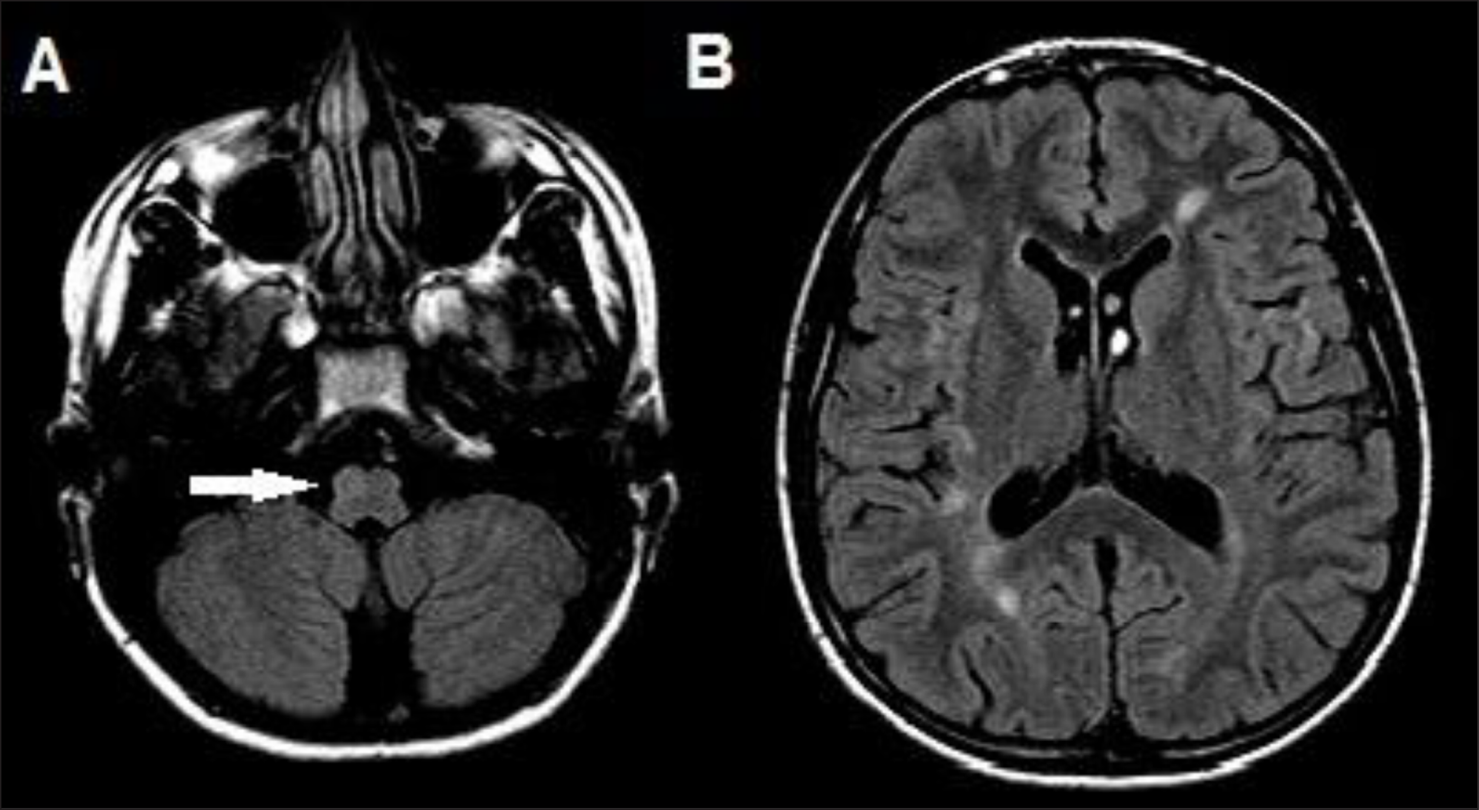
Axial FLAIR images at the level of the medulla oblongata (A) and the lateral ventricles (B) in a 36-year-old woman with established multiple sclerosis show a discrete hyperintense lesion ventrally and right-sided in the medulla oblongata (white arrow) (A). Although this lesion could reflect a hypertrophic degeneration of the right-sided inferior olivary nucleus, there were no other lesions along the Guillain-Mollaret triangle, and the patient did not exhibit symptoms typical for HOD. Due to the simultaneous presence of multiple periventricular and juxtacortical supratentoriell white matter lesions (B), a diagnosis of a demyelinating lesion was made.
